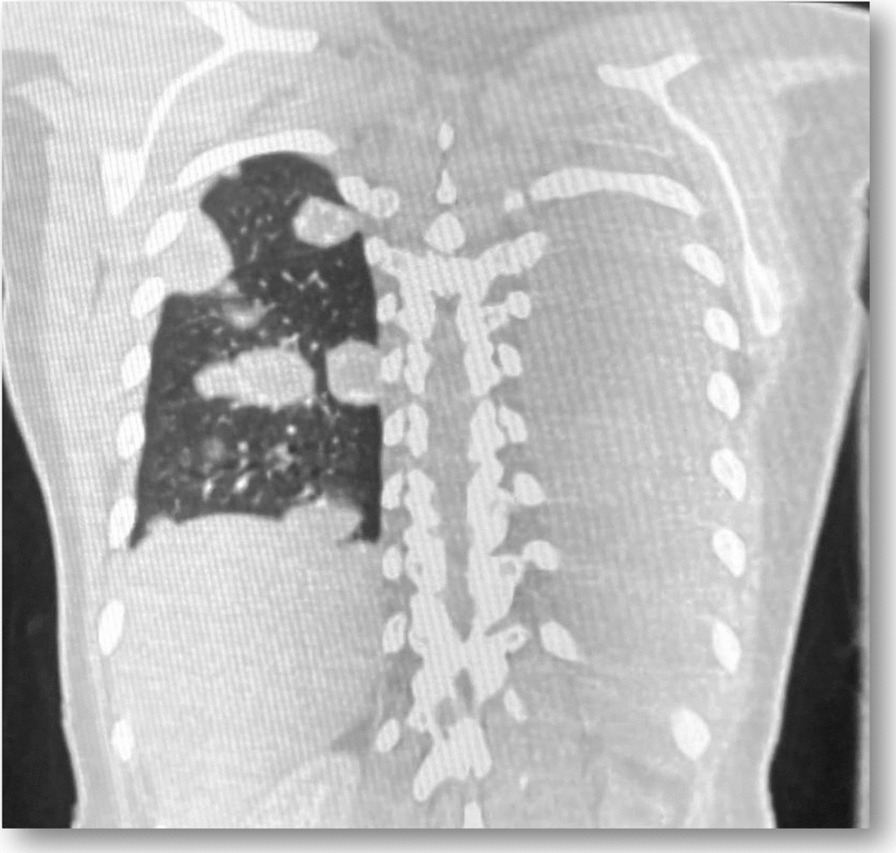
Bilateral pulmonary nodes (up to 41 mm in the right upper lobe and 34 mm in the left lung) in HRCT suggestive of metastases
